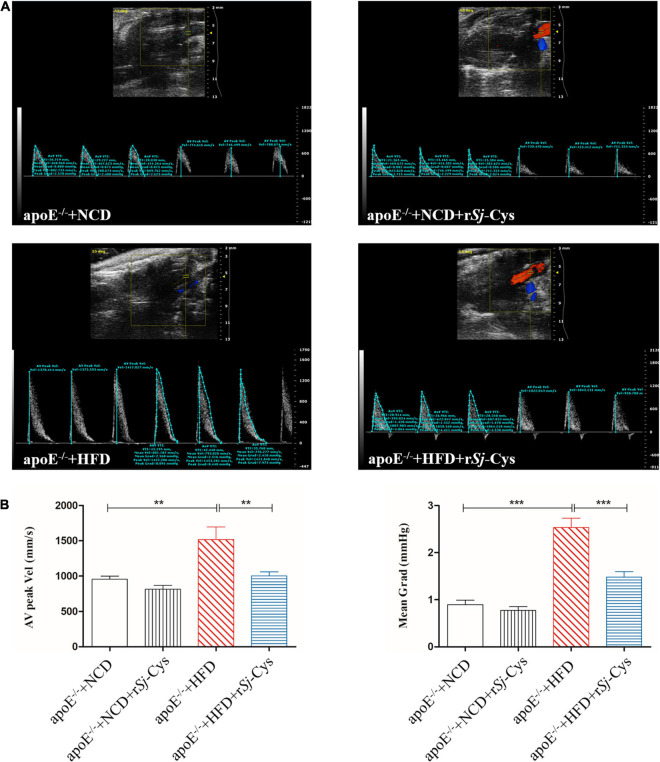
Treatment of r*Sj*-Cys improved hemodynamic function. (A) Representative color Doppler ultrasound of the heart. (B) r*Sj*-Cys improves the AV peak velocity and mean gradient of the ascending aorta. The data are shown as the mean ± SEM for each group (*n* = 6 per group). *P* < 0.01, P* < 0.001.
